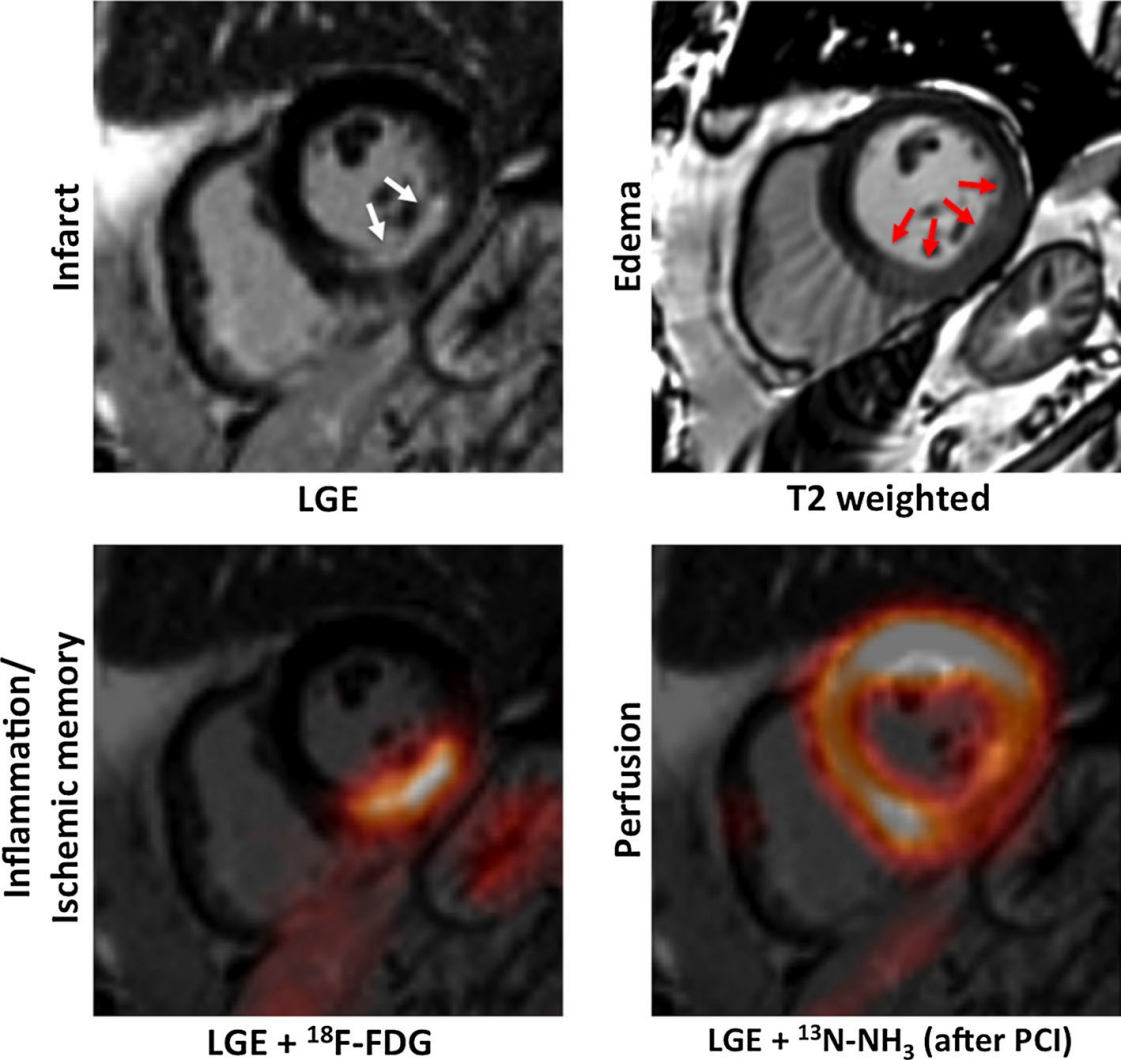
Multimodal characterization of the myocardial tissue after AMI using PET/MRI. Short-axis images of a patient who was imaged shortly after acute MI using simultaneous ^18^F-FDG and ^13^N-NH_3_ PET/MRI. Myocardial scarring can be imaged using LGE MRI (left column, top; white arrows pointing at subendocardial non-transmural infarction). The area of myocardial infarction is exceeded by the myocardial oedema imaged using T2-weighted sequences (right column, top; red arrows). Using fasting-heparin ^18^F-FDG-PET/MRI, the area of post-ischemic inflammation or ischemic memory can be assessed. After revascularization by percutaneous coronary intervention (PCI), only a slightly reduced perfusion of the inferior wall was observed in this patient [113]
